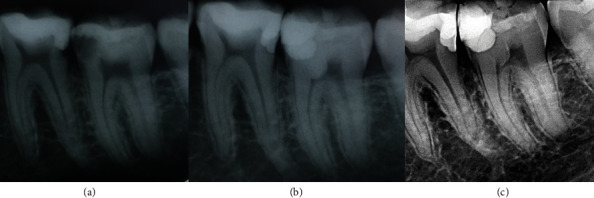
Permanent tooth 37. The image (a) shows diagnostic X-ray, image (b) immediate postoperative, and image (c) control at 25 months.
